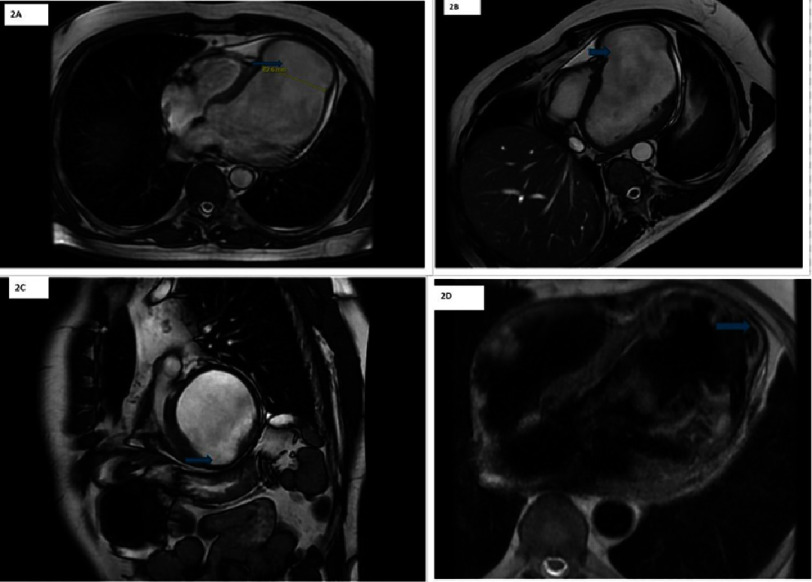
Cardiac MRI showed an aneurysm with a maximal diameter of 7 cm (2A) and a neck diameter of 6.5 cm (2B and 2C), suggesting a left ventricular pseudoaneurysm. Layering of thrombus within the aneurysm is observed (2D), raising concerns for pseudoaneurysm formation.
